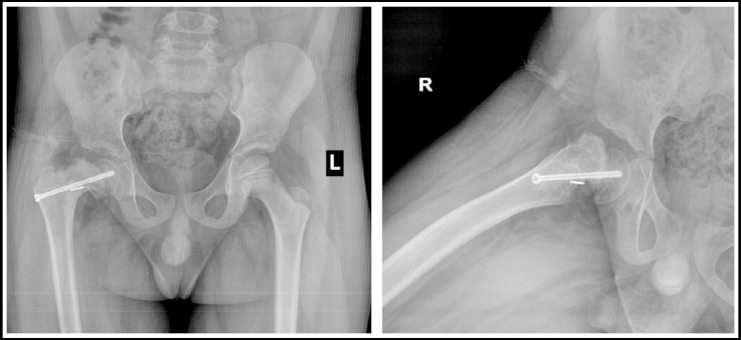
X-rays after fixation of slip with cannulated screw.
